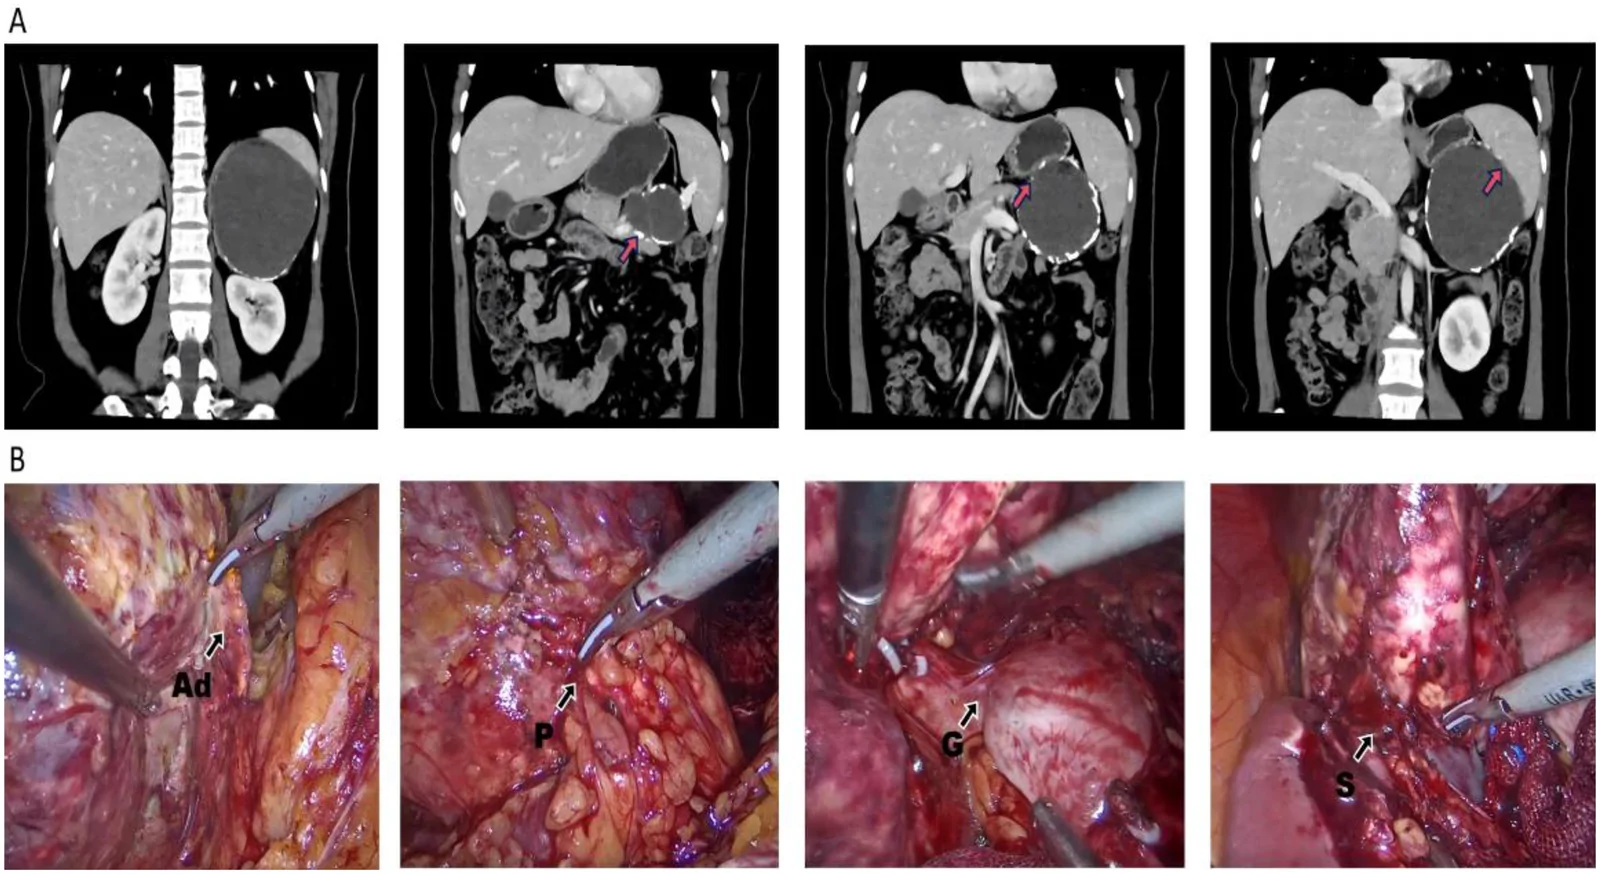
(A) Contrast-enhanced abdominal CT showed that the left adrenal gland was not distinctly identified. A giant cystic low-density lesion was seen in the left retroperitoneum, closely related to the stomach, spleen, and pancreas, with compression and displacement of the left kidney and no obvious enhancement on contrast-enhanced imaging. The arrows in the second, third, and fourth images indicate the interfaces between the lesion and the pancreas, stomach, and spleen, respectively. (B) Intraoperative images showing the adhesions between the giant left retroperitoneal cyst and adjacent organs. From left to right, Ad, adrenal gland; P, pancreas; G, stomach; and S, spleen. The arrows indicate the sites of adhesion between the cyst and the corresponding adjacent organs.
